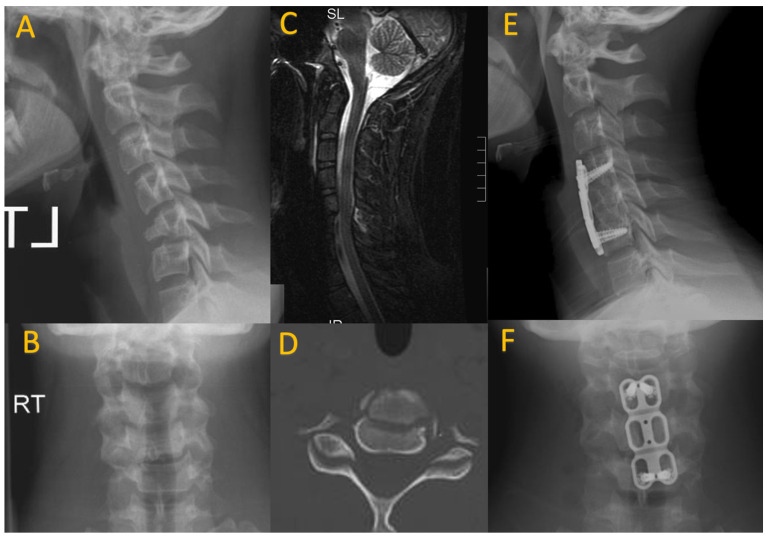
A 14-year-old male who presented after hitting a tree while snowboarding. On presentation he complained of transient weakness and persistent neck pain. He was found to have a C5 teardrop fracture on sagittal and AP cervical spine X-rays (A,B). There was interspinous ligament injury noted on the sagittal MRI STIR sequence (C). CT axial imaging again demonstrated the anterior column fracture (D). He underwent C5 corpectomy with anterior C4–6 instrumentation and allograft interbody strut (E,F).
